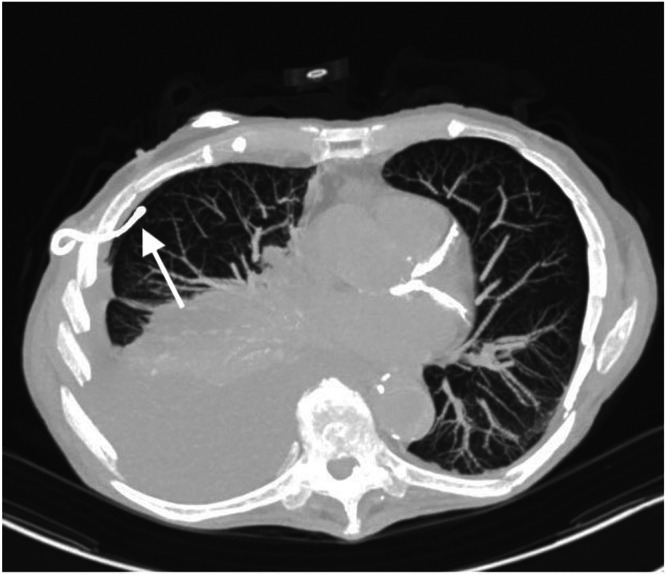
Case 2 axial computed tomography scan image showing hypoglossal nerve stimulator respiratory sensing lead intrapleural. The arrow indicates the respiratory sensing lead location.
